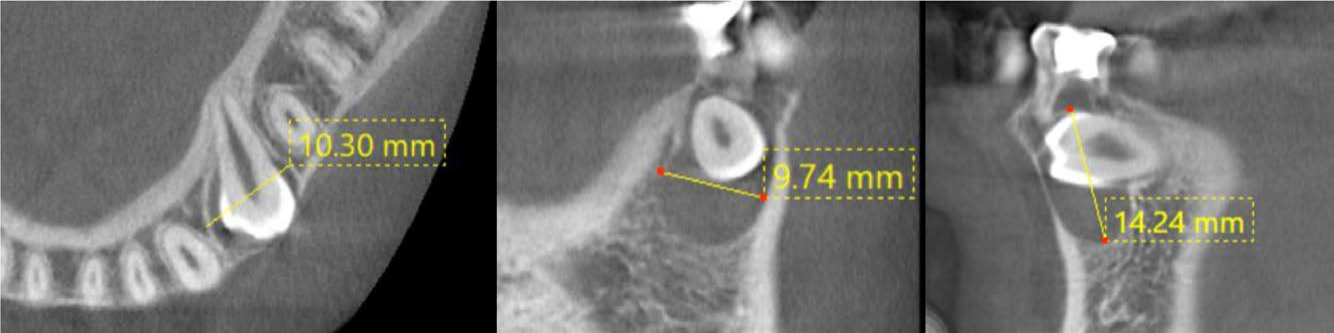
Pretreatment computed tomography image of the mandibular left first premolar.
